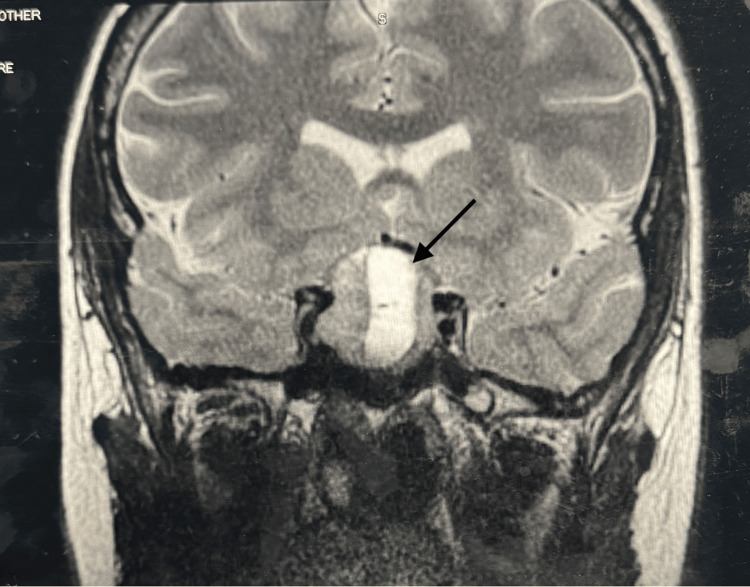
MRI of the pituitary on admission showing recurrent pituitary macroadenoma (29 × 29 × 27 mm) with central hemorrhagic component, compression of the optic chiasm, suprasellar cistern extension, and projection into the right cavernous sinus. MRI: magnetic resonance imaging
